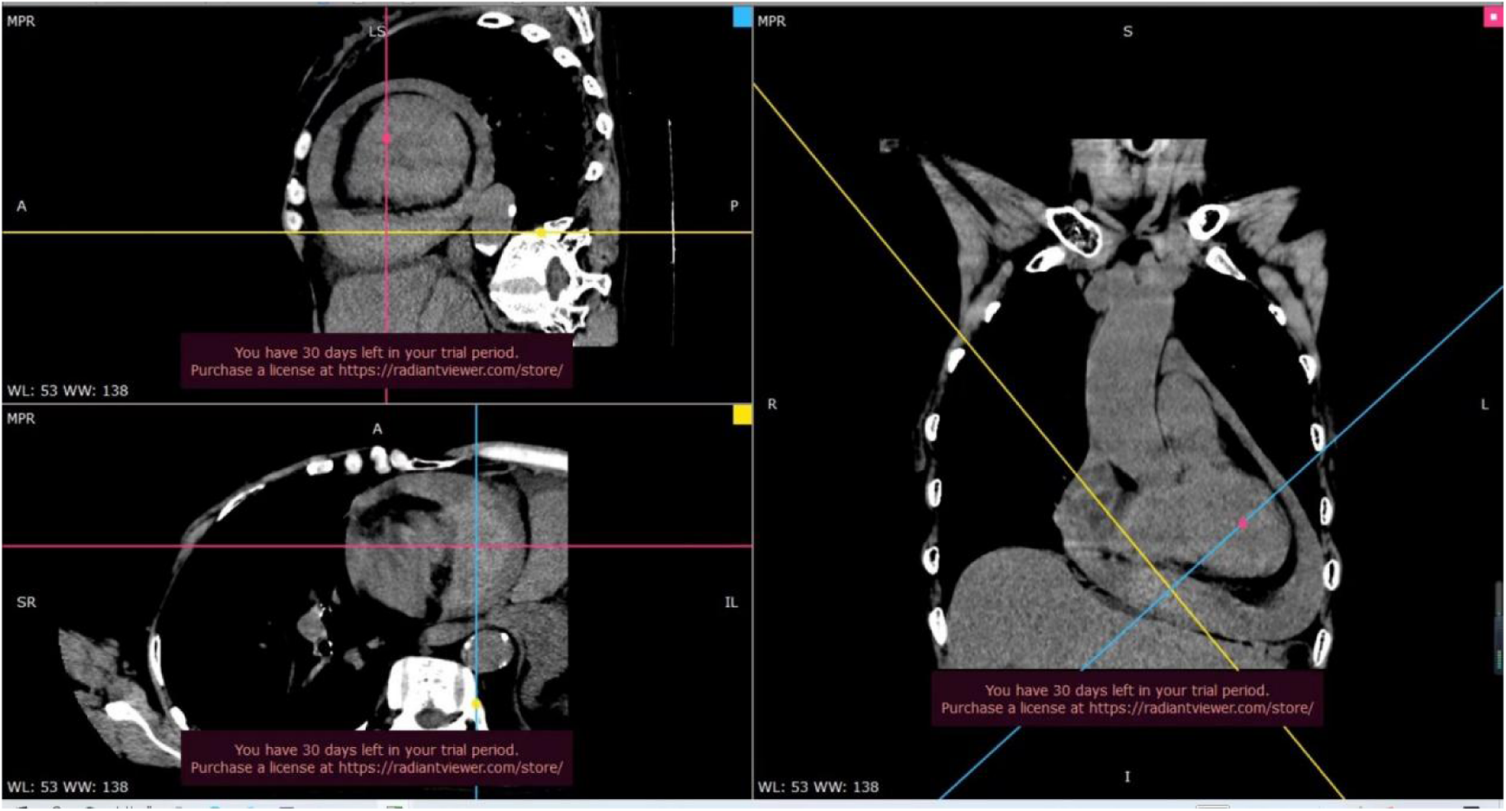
On thoracic aortic CT, a widespread slightly low-density shadow was observed in the pericardial cavity, with a CT value of approximately 30–36 hounsfield units (Hu), indicative of hemorrhagic pericardial effusion. Additionally, a mass tissue with slightly high-density shadow was noted in the pericardial cavity above the diaphragm, with a CT value of about 57 Hu. The boundary of this mass was unclear, and no significant enhancement was observed after contrast-enhanced scanning.
